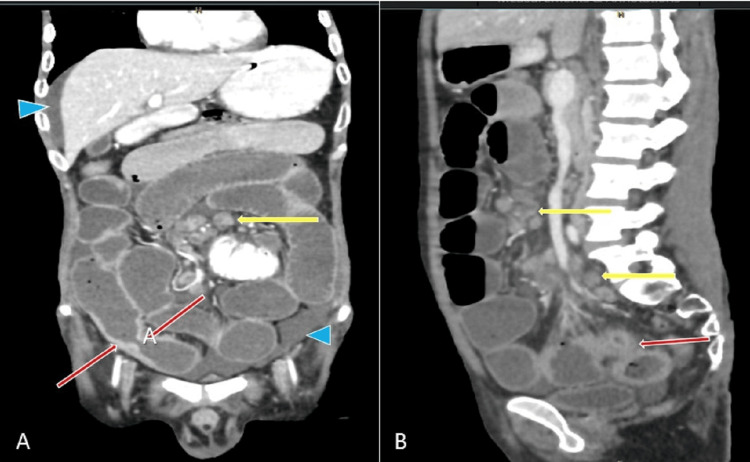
Contrast computed tomography scan, coronal and sagittal views Image A: Contrast computed tomography scan coronal image showing abdominal lymphadenopathy (yellow arrow), small bowel stricture (red arrows), and ascites (blue arrowheads). Image B: Contrast computed tomography scan sagittal image showing abdominal lymphadenopathy (yellow arrows) and small bowel stricture (red arrow).
